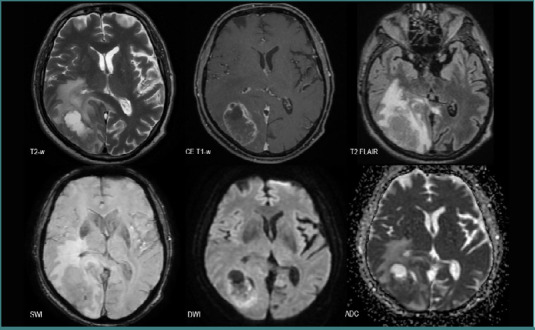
MRI brain acquisition of a patient with squamous cell carcinoma. Voluminous right parieto-occipital lesion, with important central necrosis and cystic component (the area with high T2-w, low CE T1-w signals and facilitated diffusion – low DWI and high ACD signals). It also displays poor intralesional vascularization (a small spot on SWI), significant peripheral edema (high T2-w and T2-w FLAIR signals), and diffusion restriction of the remaining solid component (high DWI and low ADC).
